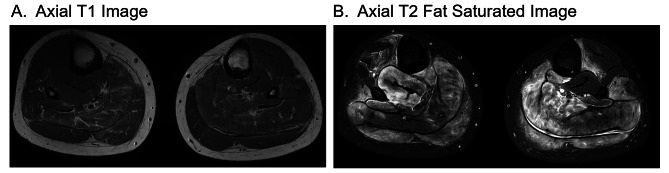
Inflammation, atrophy, and fasciitis on lower limb MRI. An axial T1 image (A) demonstrates increased intramuscular fat signal bilaterally consistent with atrophy. An axial T2 fat saturated image (B) demonstrates diffuse muscular edema in all four compartments
